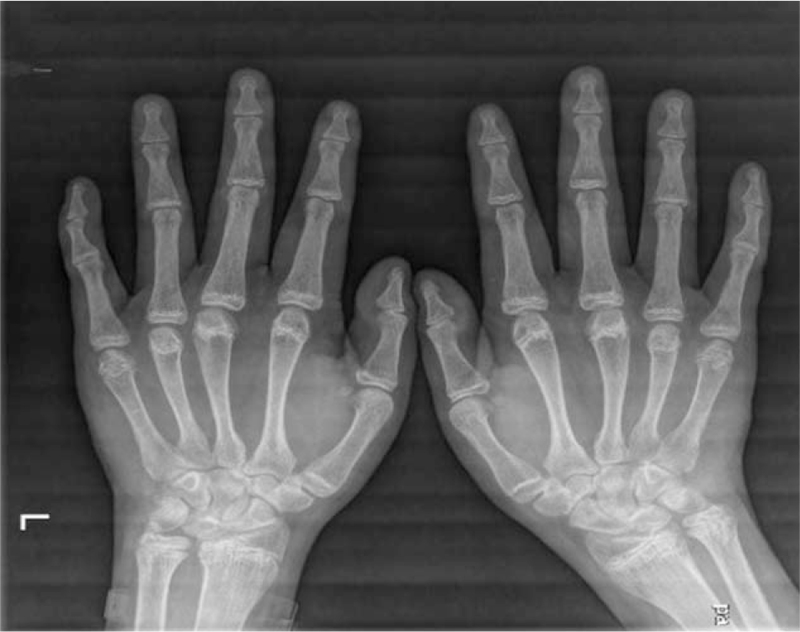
x-Ray examination of both hands demonstrates that the bone age of the patient is 16 years.
